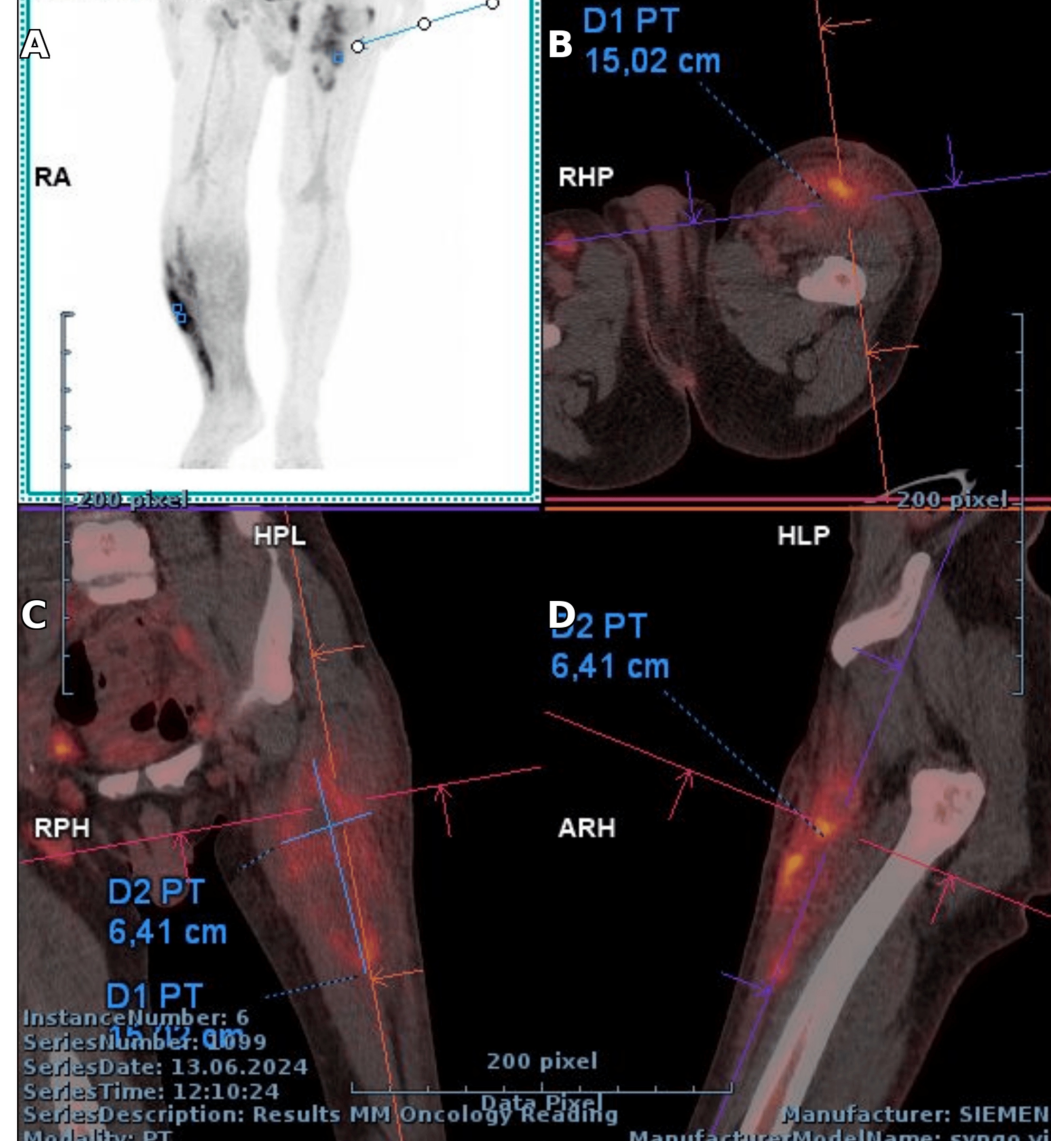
PET/CT of the right lower limb with volumetric analysis: (A) MIP showing two hypermetabolic areas in the right leg and left thigh; (B) Ax slice with lesion 15.02 cm (D1 PT); (C) Cor slice with lesion 6.41 cm (D2 PT); (D) Sag slice confirming both lesions (D1 PT, D2 PT).
